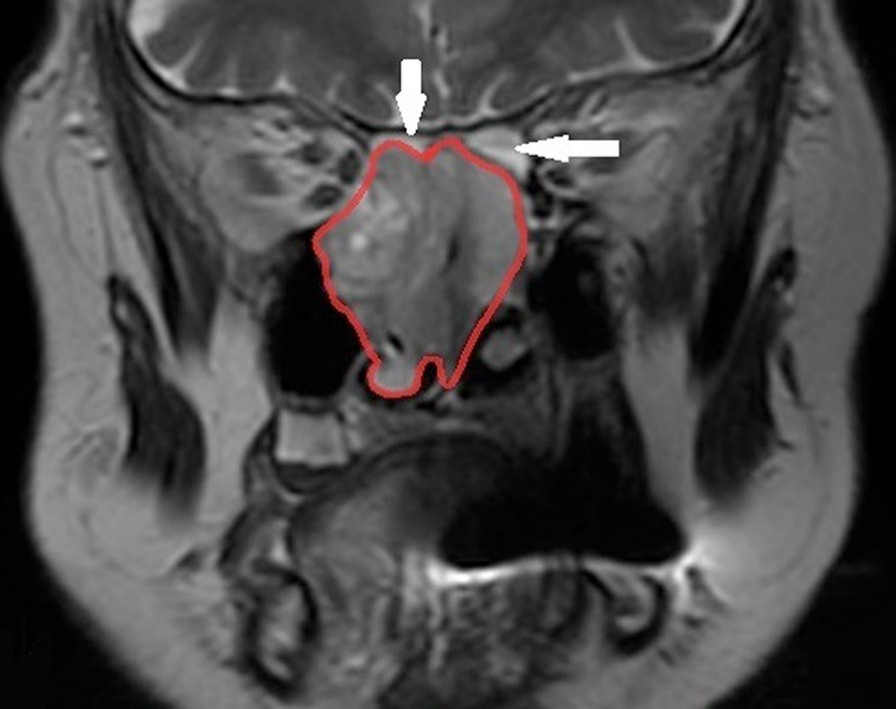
Magnetic resonance imaging. Coronal projection. Tumor masses in the right nasal cavity and bilaterally ethmoid sinuses. The *arrows* indicate ethmoids in inflammatory state, separating the tumor from anterior cranial fossa
